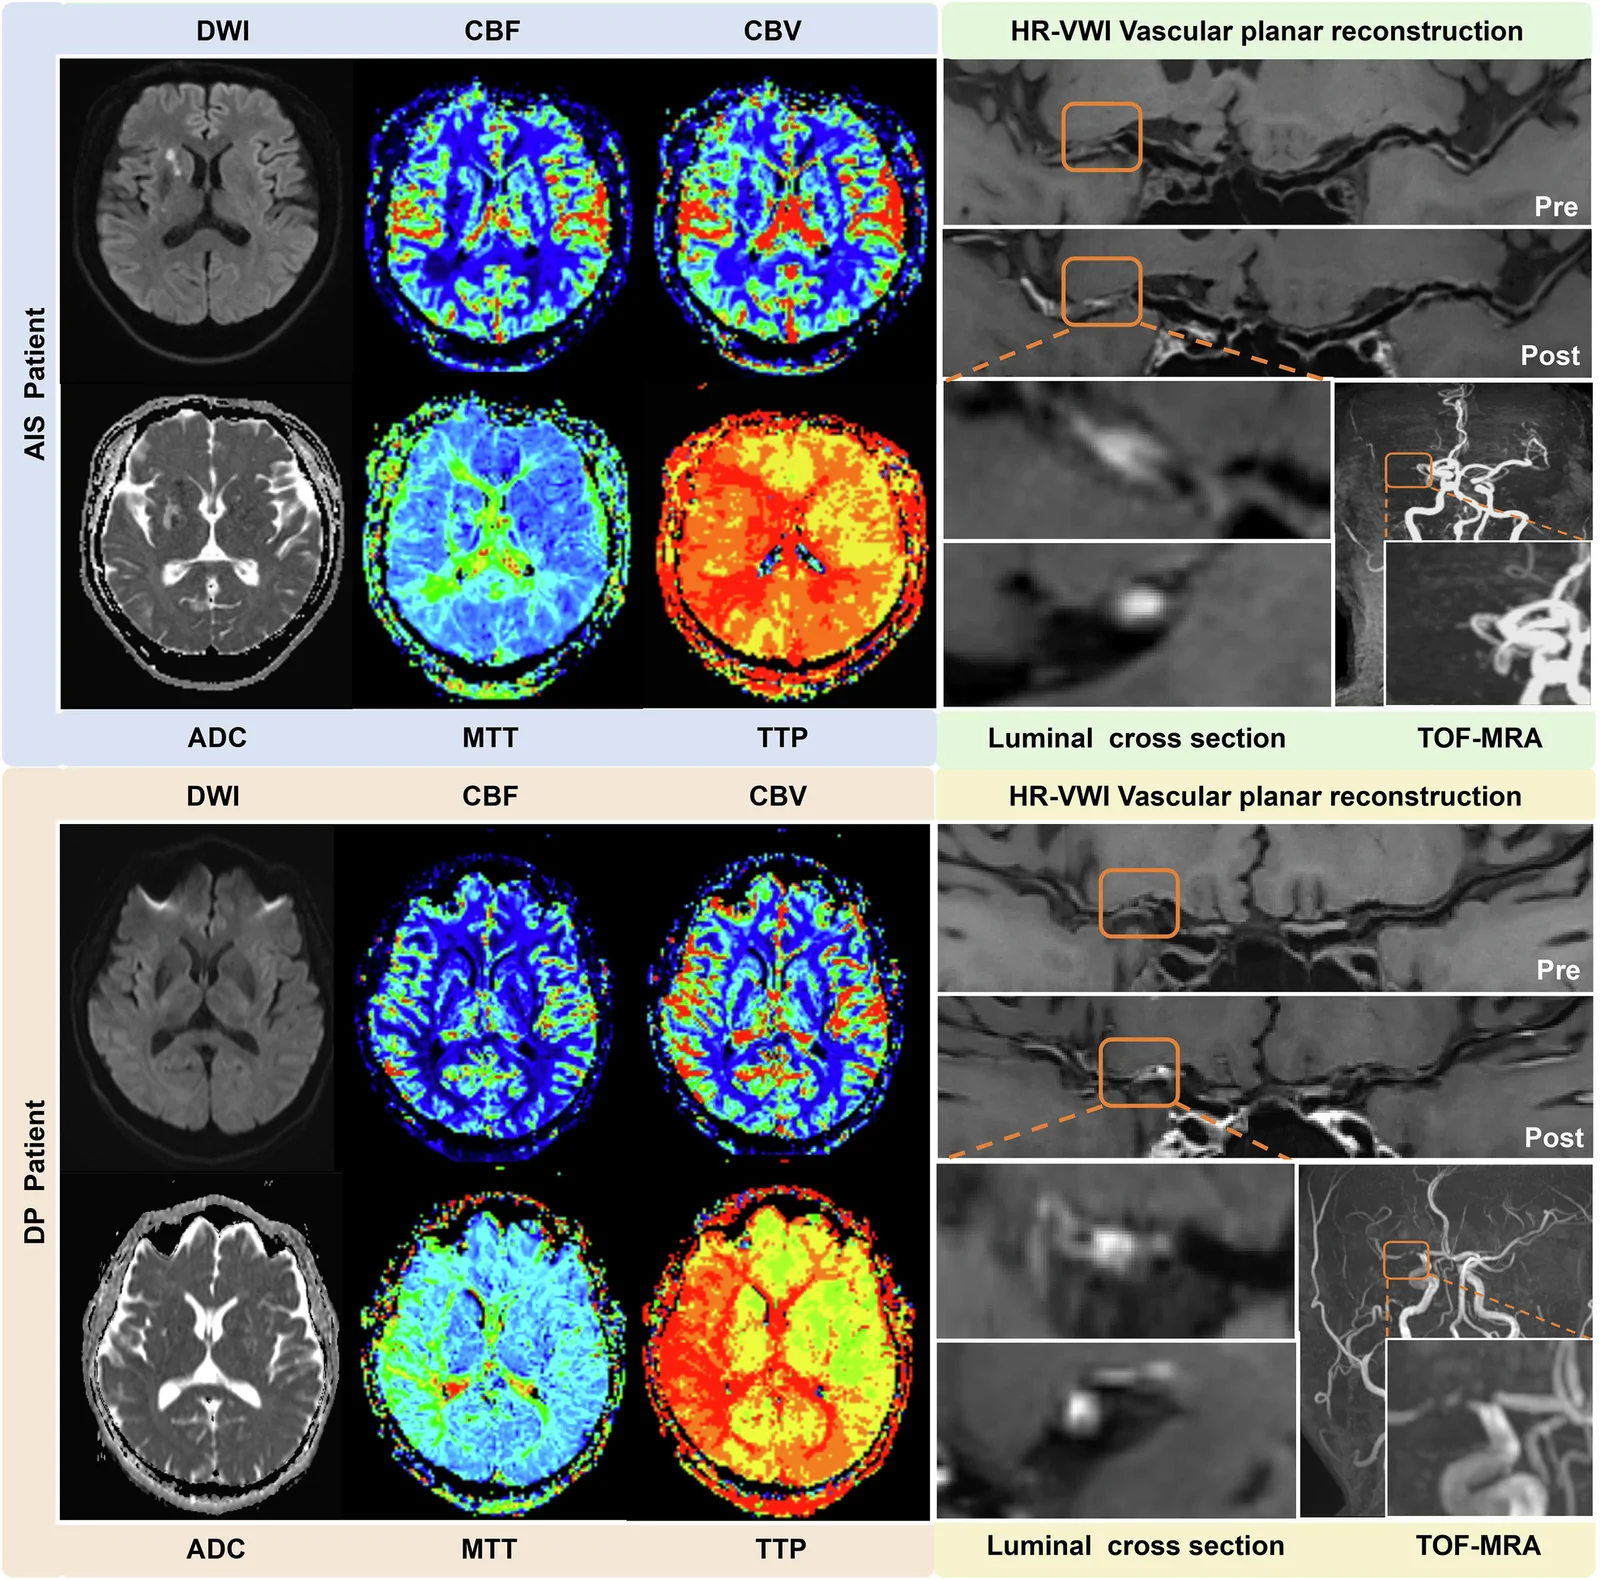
Example of patients’ images for acute ischemic stroke and delayed perfusion. AIS patients were identified by the presence of patchy hyperintensity on DWI with correspondingly reduced ADC values. Their PWI showed decreased CBF and CBV alongside prolonged MTT and TTP in the ischemic area. HR-VWI revealed a high-risk plaque in the right middle cerebral artery, characterized by pre-contrast intraplaque hyperintensity (suggesting hemorrhage) and marked post-contrast enhancement. This plaque caused local luminal occlusion and downstream segmental occlusion, warranting a Plaque-RADS 4 classification. DP patients were defined by the absence of DWI/ADC abnormalities. However, PWI demonstrated prolonged MTT and TTP in the symptomatic region. HR-VWI also identified a high-risk plaque in the same artery. It appeared irregular pre-contrast and showed marked enhancement post-contrast, resulting in severe local luminal stenosis while the downstream vessel remained patent. This plaque was also classified as Plaque-RADS 4. DWI, diffusion-weighted imaging; ADC, apparent diffusion coefficient; PWI, perfusion-weighted imaging; CBF, cerebral blood flow; CBV, cerebral blood volume; MTT, mean transit time; TTP, time to peak
